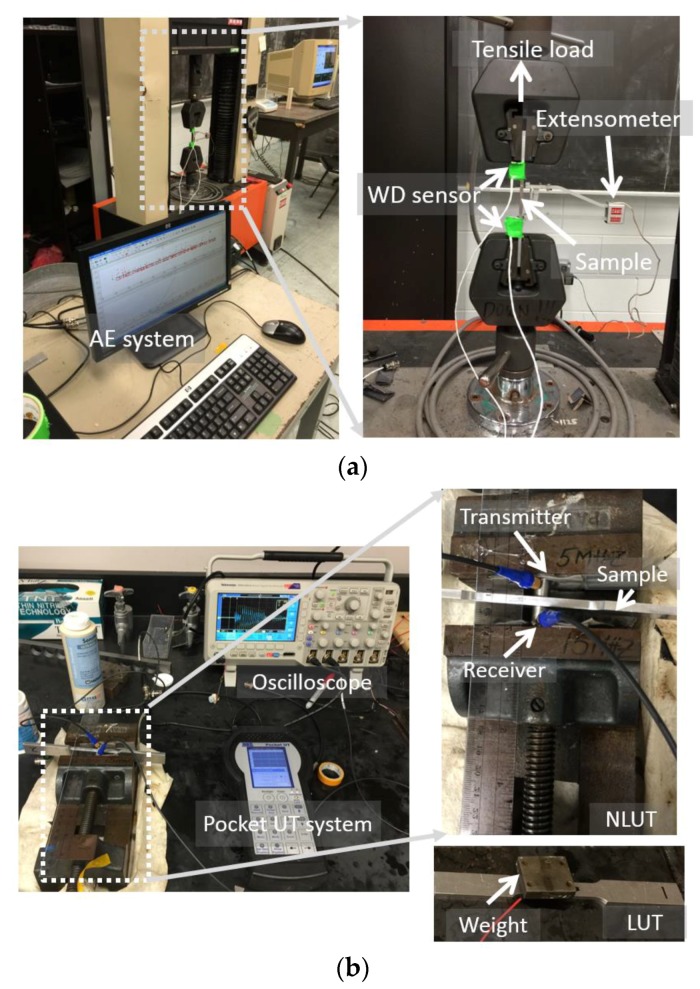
The experimental setups; (a) tensile test and acoustic emission (AE) setup and (b) ultrasonic testing (UT) measurements including linear ultrasonics (LUT) and nonlinear ultrasonics (NLUT).
